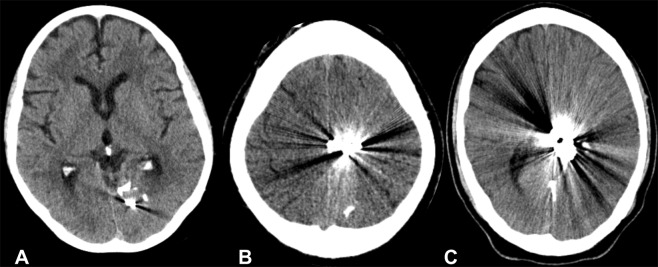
Un-enhanced CT scans of the brain after embolization of Arteriovenous malformations (AVMs) with Onyx, in the left occipital lobe (A), in the left parietal region (B) and left parietotemporal region (C). Noticeable are the artifacts that reduce diagnostic image quality.
